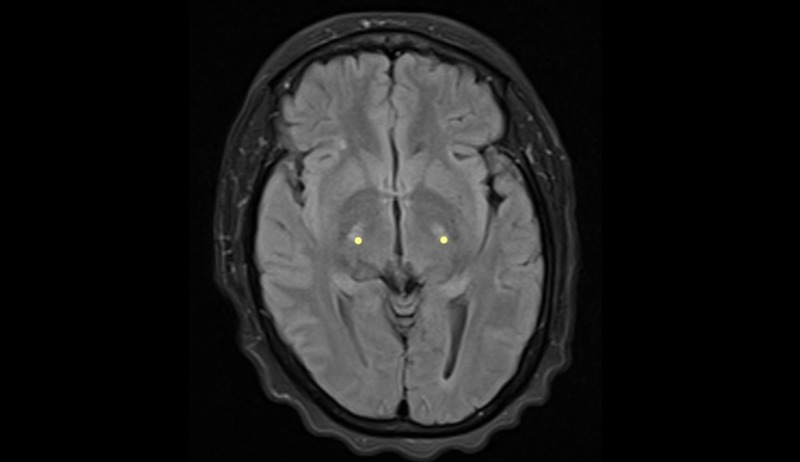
Brain MRI - view 2 FLAIR axial and T2-weighted coronal image. The image shows hyperintensity in the path of the corticospinal tract (yellow dots) MRI: magnetic resonance imaging; FLAIR: fluid attenuation inversion recovery
